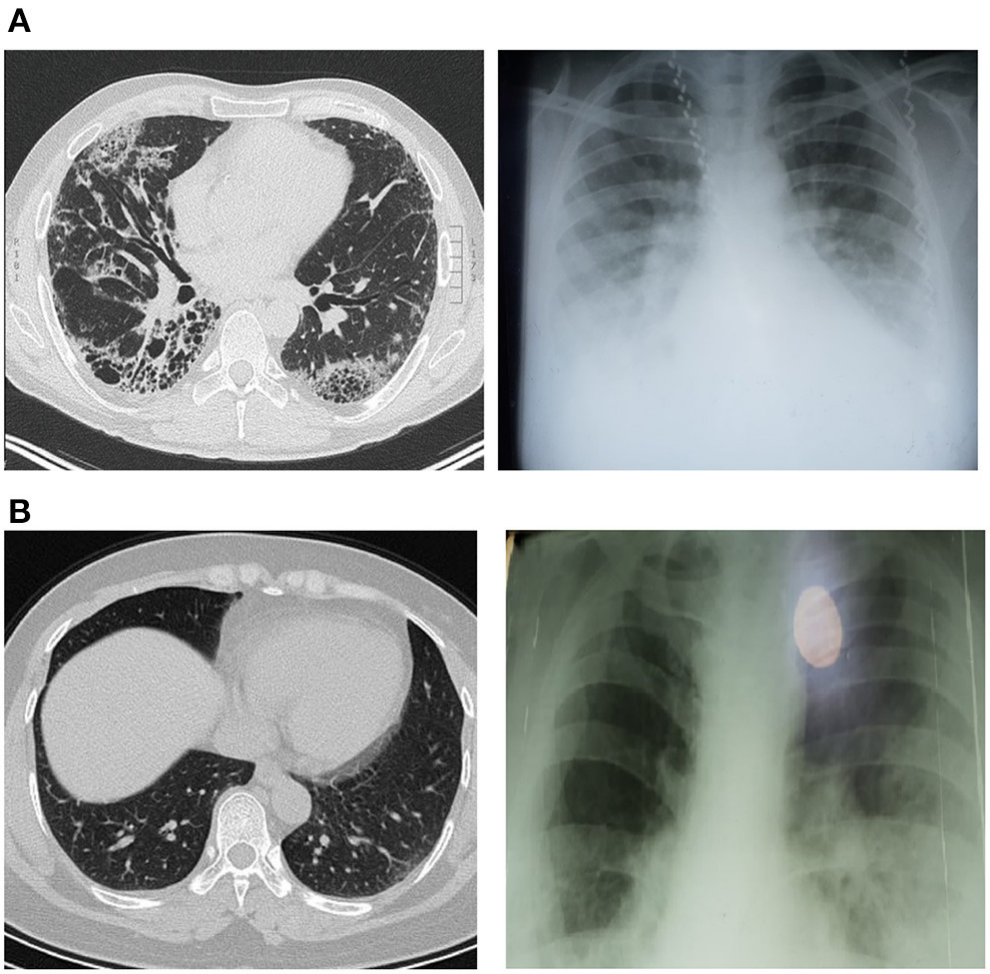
Chest X-ray and CT scan imaging showed bilateral ground-glass appearance; (A) Covid-19 patients only, (B) Covid-19 patients with Gilbert syndrome.
